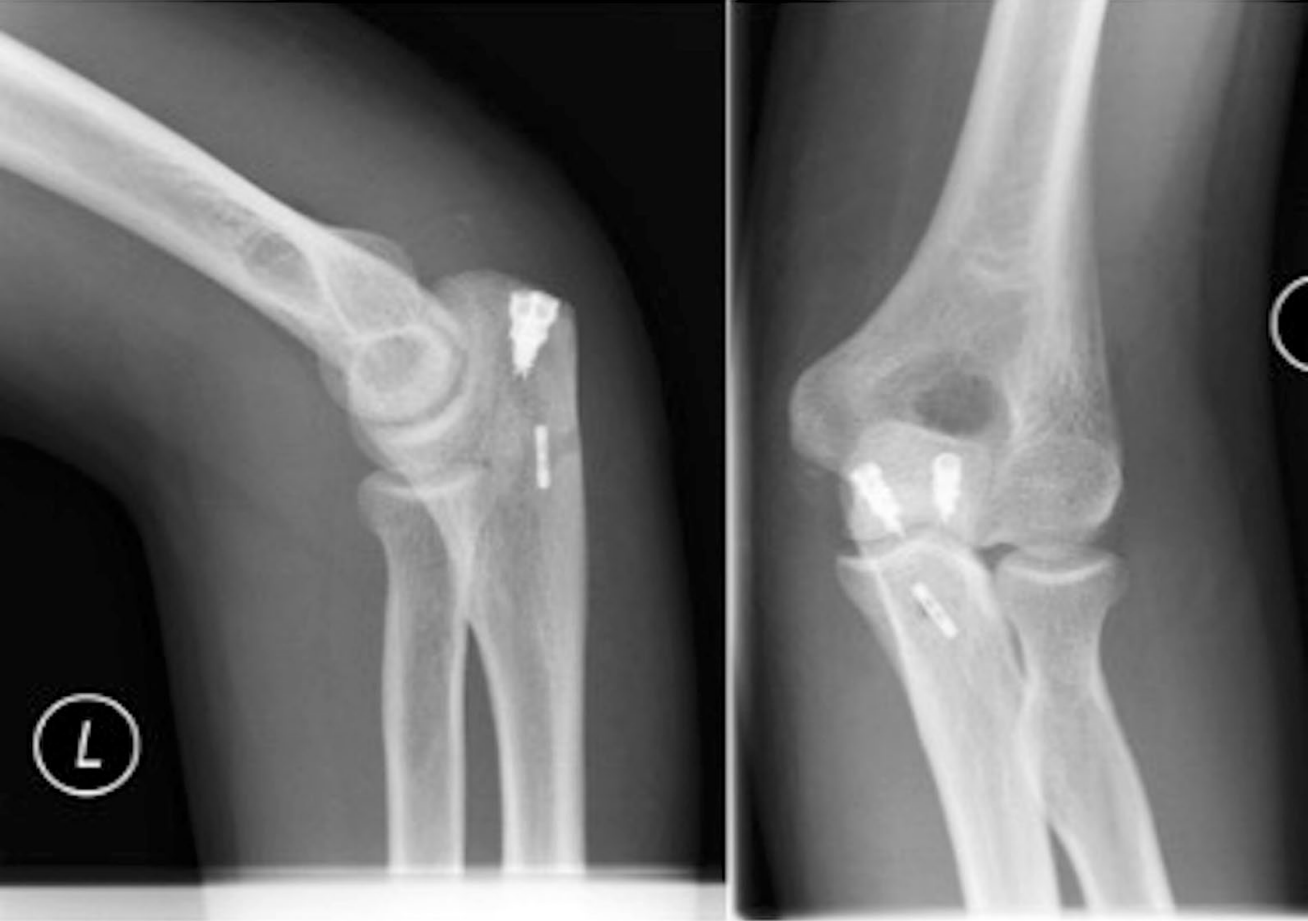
Postoperative radiograph showing the intramedullary cortical button
